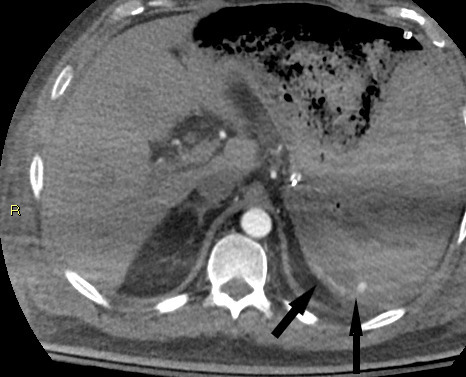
Contrast-enhanced CT of the upper abdomen shows active contrast extravasation (black arrows) from the fundal gastric wall consistent with active bleeding.
